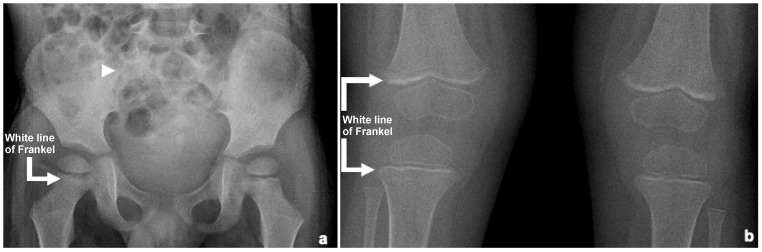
Radiological images from a three-year-and-nine-month-old boy with scurvy. (a) An anteroposterior hip radiograph shows no abnormalities in the sacroiliac joints (arrowhead). A mild dense band is observed in the metaphysis of the proximal femur (arrow). Superim position of bowel meteorism is also noted; (b) An anteroposterior knee radiograph reveals increased density in the metaphyses of the distal femur and proximal tibia bilaterally (arrows). A diffuse reduction in bone mineral density is also present.
